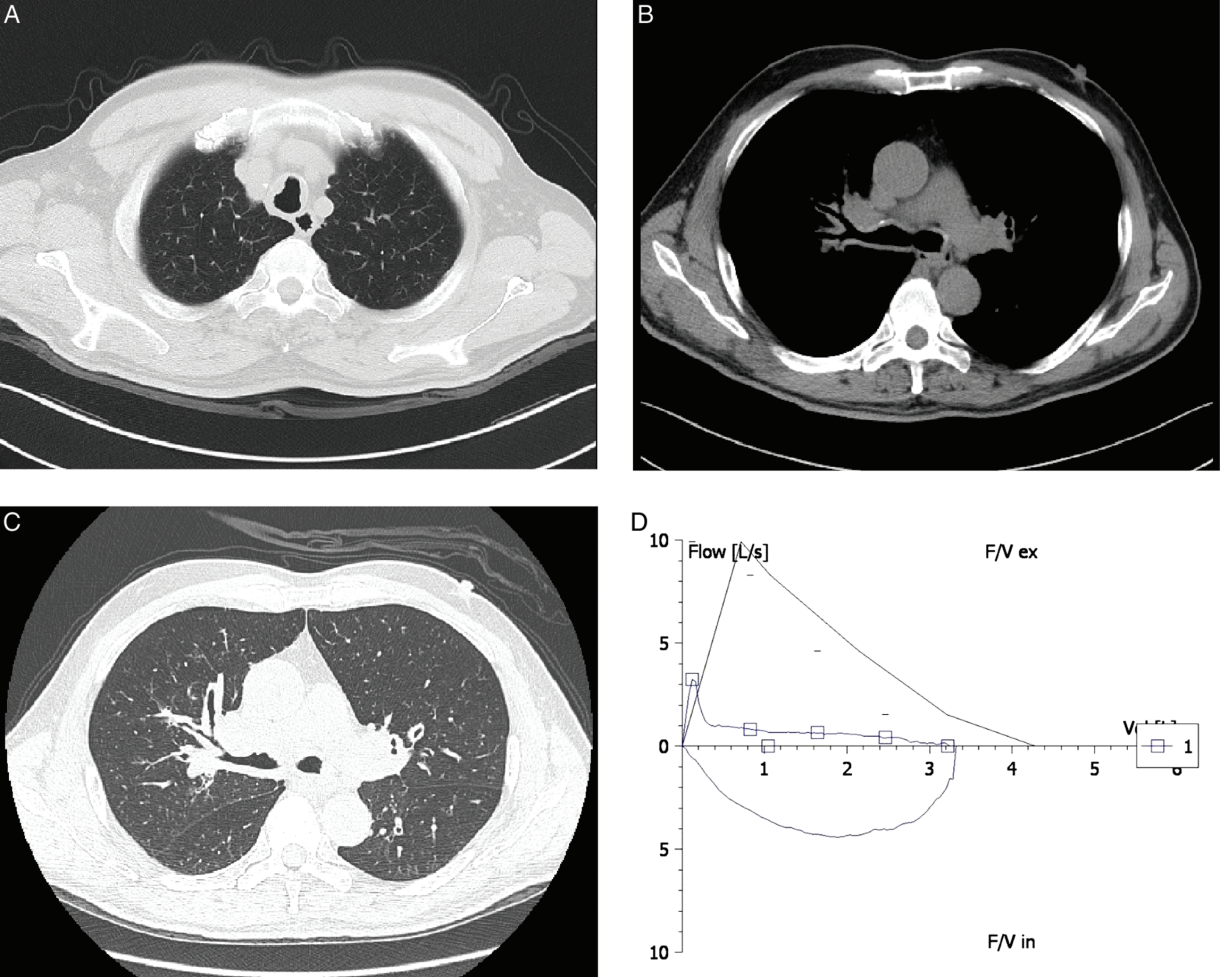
Non‐contrast chest computed tomography scan showing irregular tracheal wall thickening (A) and bilateral bronchial wall thickening (B, C). Pulmonary function testing showing obstructive ventilatory dysfunction (D).
